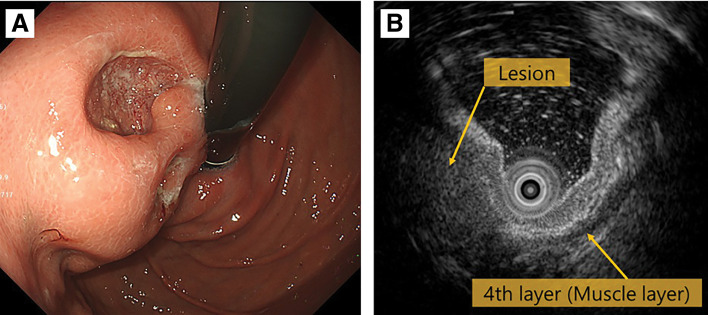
The upper gastrointestinal examination and the endoscopic ultrasound examination images. (A) There was a submucosal tumor with an ulcer just below the gastric cardia. (B) Endoscopic ultrasound revealed a hypoechoic mass with irregular margins just below the cardia, suggesting a tumor of muscularis.
